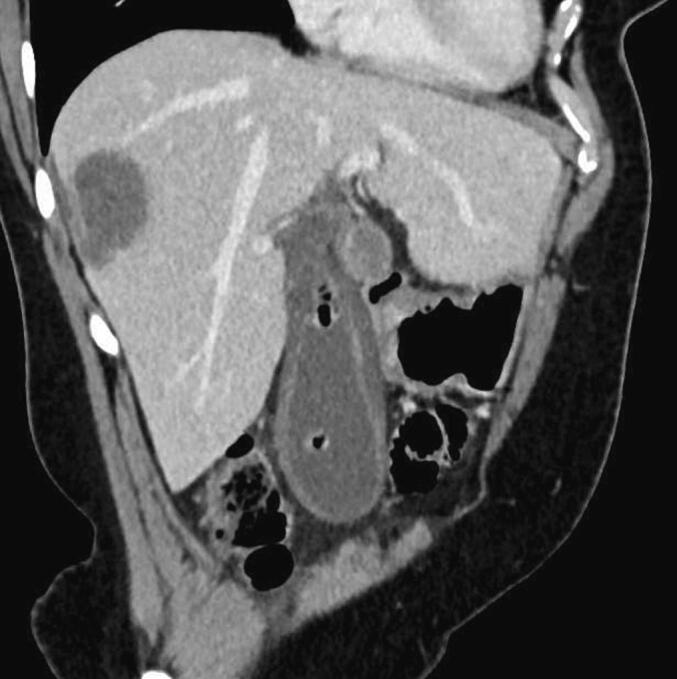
CT images showing the distended gallbladder with a thick wall and a gas in the gallstones.
